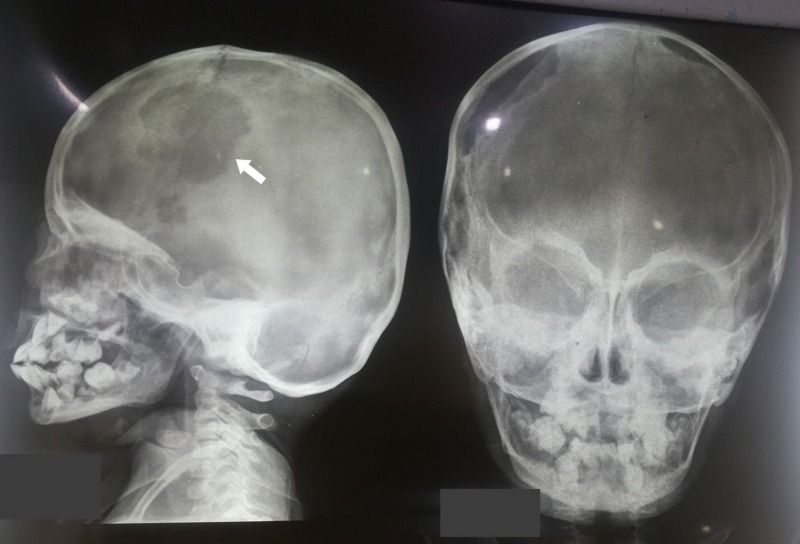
X-ray showing lytic lesion in the skull.
